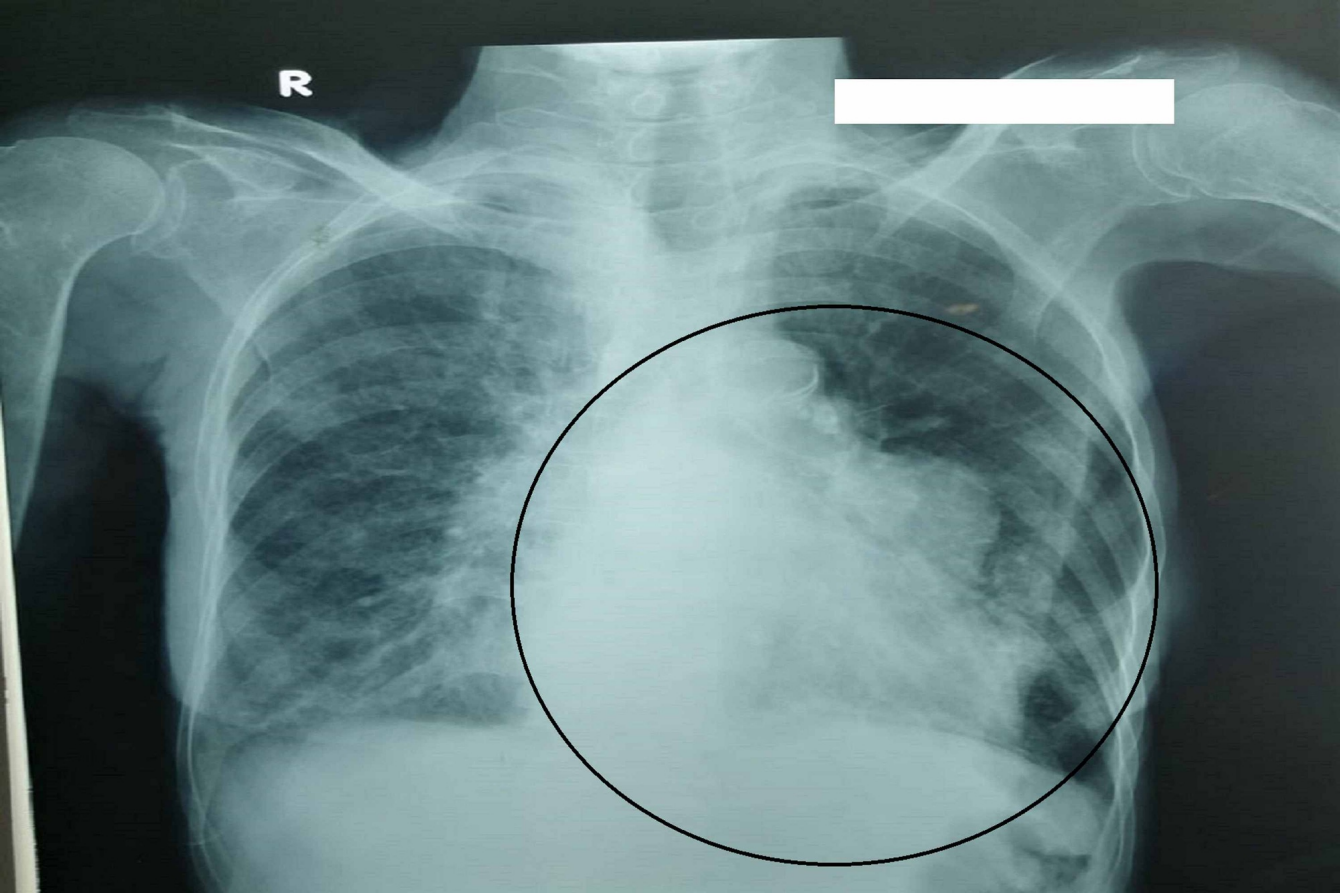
Chest X-ray showing cardiomegaly along with irregular left cardiac border mimicking homogenous opacity in left mid-zone of the lung.
